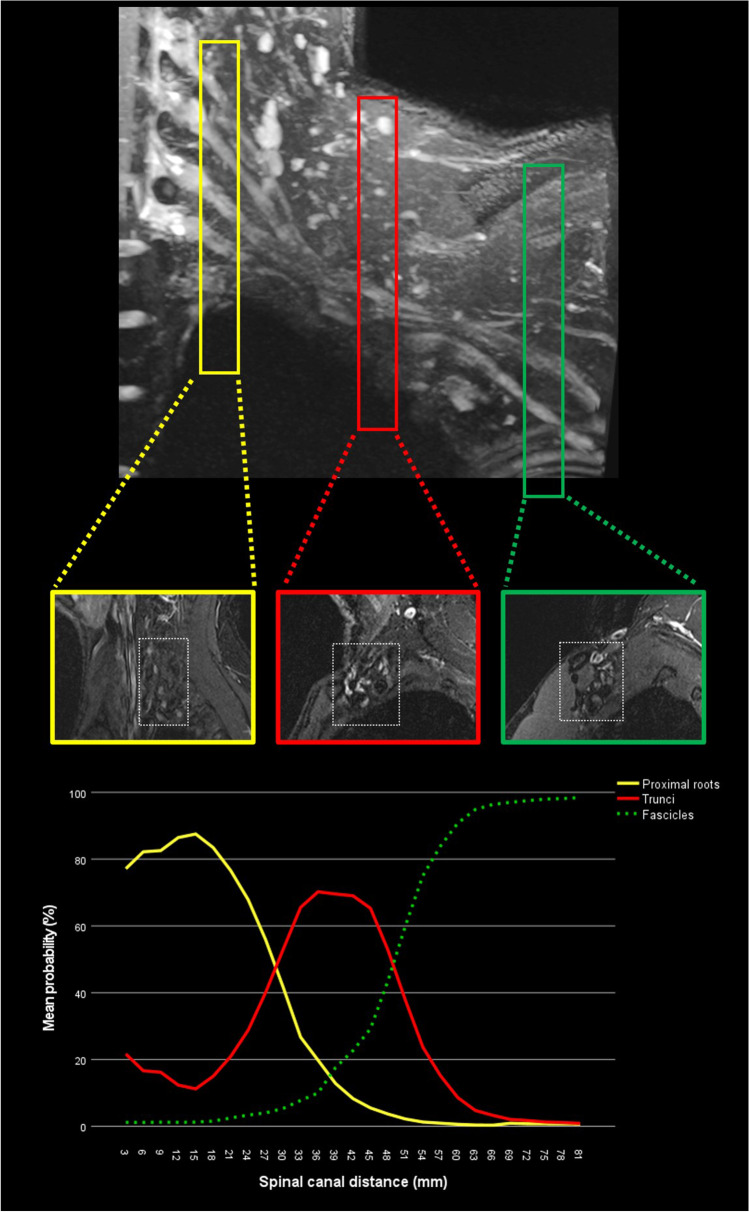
Algorithm performance expressed as probabilities of nerve position as either root, trunci, or fascicles in sagittal MR images of the brachial plexus (own unpublished data). Image numbers increase from medial to lateral, beginning at the cervical spine (3-mm slice thickness)
